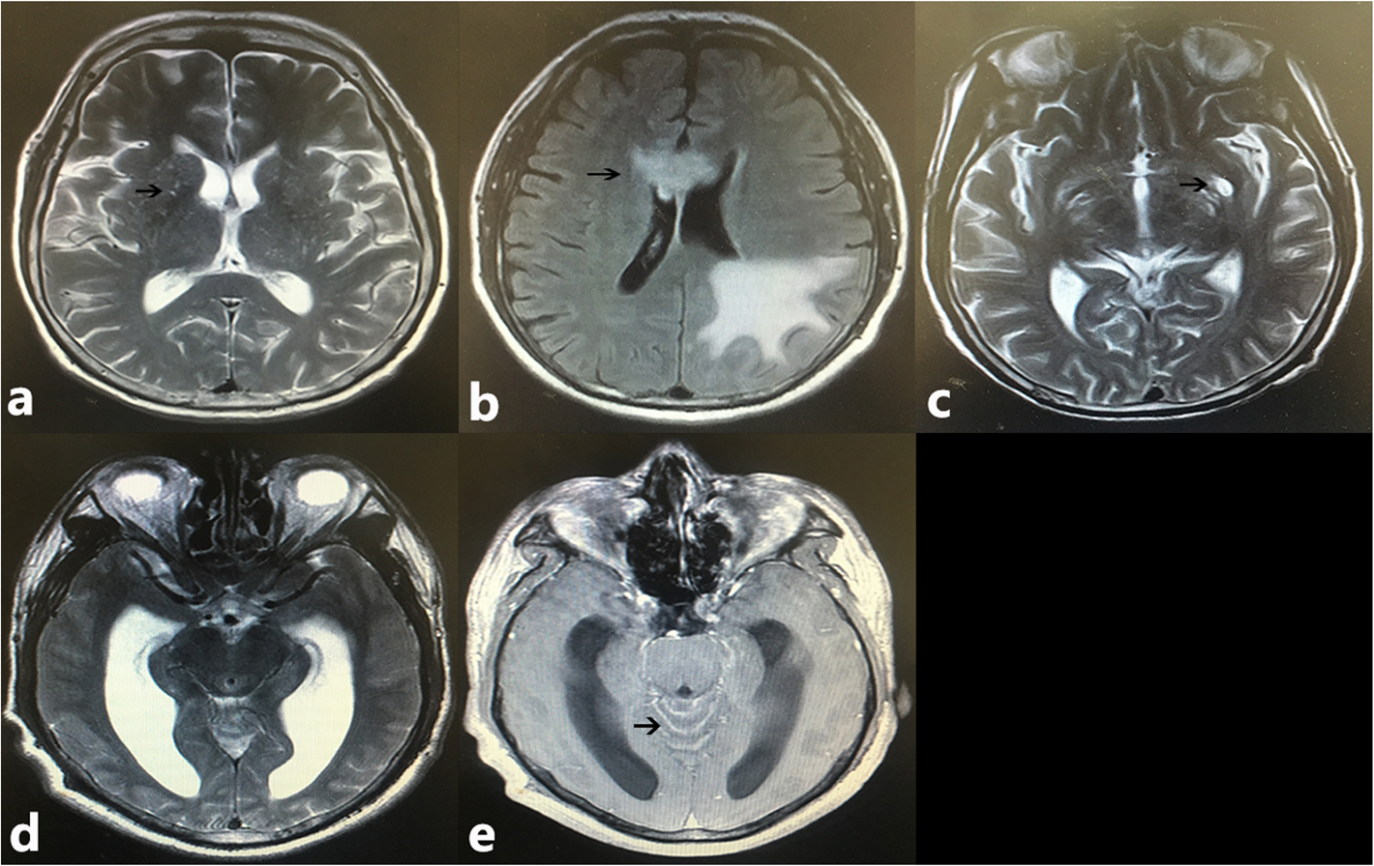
Neuroimaging characters of patients with cryptococcal meningitis. a T2-W image shows multiple dilated Virchow-Robin spaces (black arrow) in basal ganglia; b Abnormality (black arrow) on FLAIR image within the occipital lobe and corpus callosum; c T2-W image shows a gelatinous pseudocyst (black arrow) in basal ganglia; d hydrocephalus on T2-W image; e Contrast-enhanced image shows meningeal enhancement (black arrow) in cerebellum
